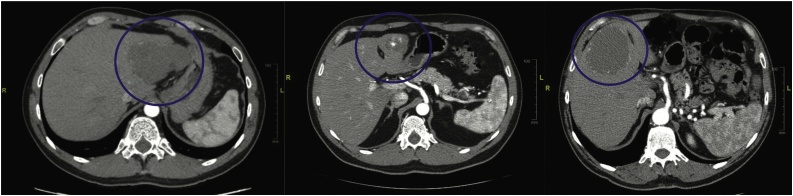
Pre operatory CT scan showing liver hemangioma. Legend: Case 1 CT scan (left); Case 2 CT scan (center); Case 3 CT scan (right).
